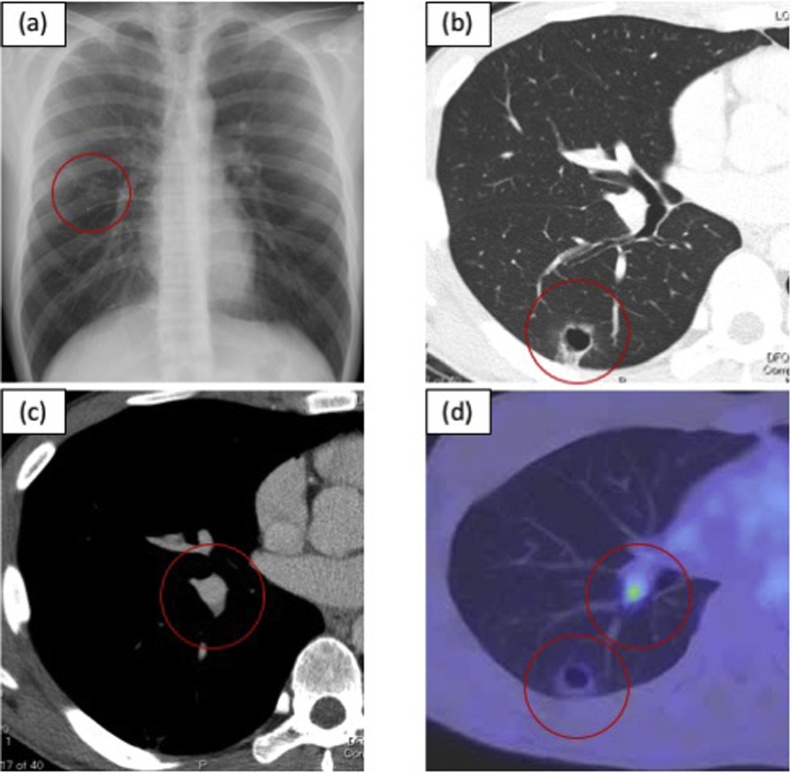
a Chest X-ray showed a cavity-lesion in the right middle field. b CT showing a GGO-like lesion with a thin-walled cavity. c Contrast chest CT showing no finding of an abnormal hilar lymph node metastasis. d PET examination showing SUV up take in the lesion with the thin-walled cavity and hilar lymph node.
